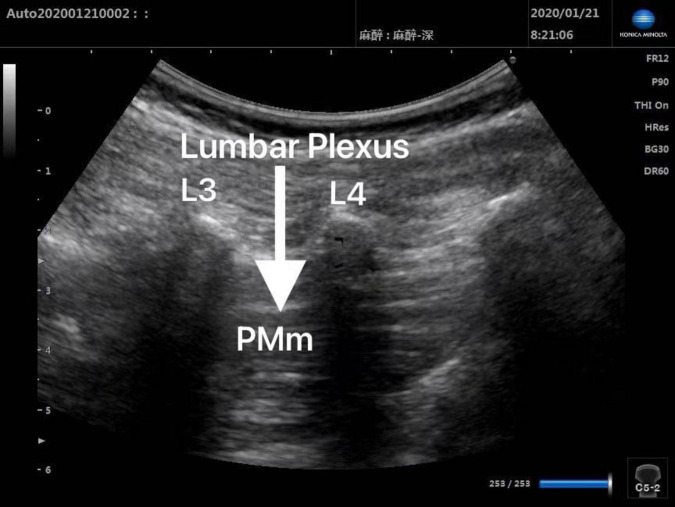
Preinjection ultrasound image of L3-4 lumbar plexus block.
